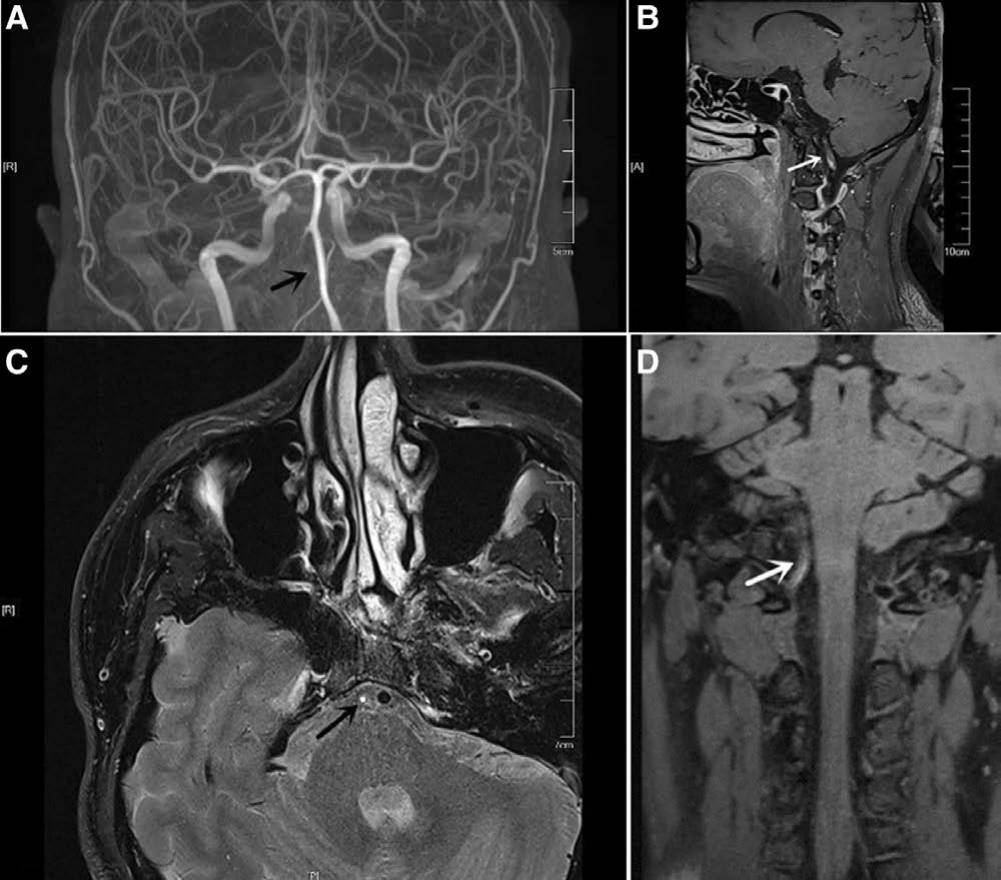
Magnetic resonance angiography scans of the head display occlusion of the right vertebral artery (A). High-resolution vessel wall magnetic resonance imaging reveals intramural hematoma (B) in the right vertebral artery. The hematoma exhibited high signal intensity on T1-weighted images (C). Arterial wall thickening with severe luminal narrowing was observed (D).
